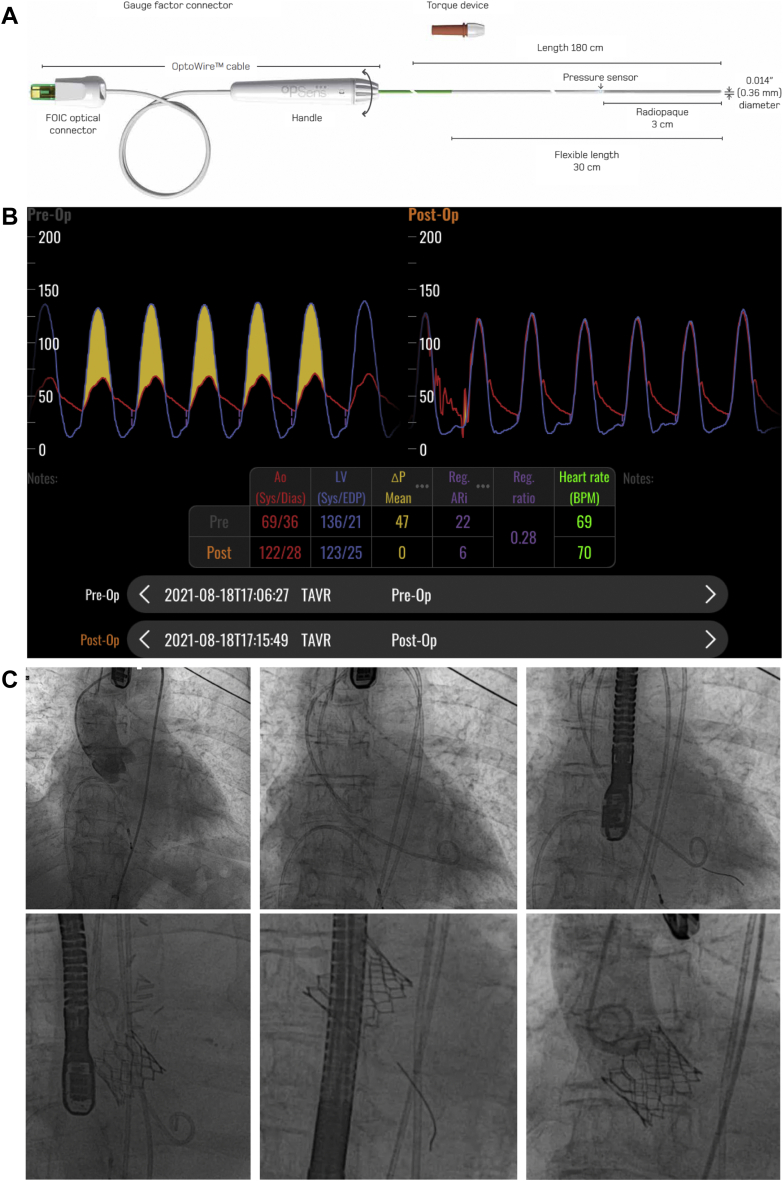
OpSens OptoWire III, monitor display, and procedure steps. (A) OpSens OptoWire III 0.014″; (B) monitor displaying pre- and post-transcatheter aortic valve replacement (TAVR) measurements; (C) summary of the procedure. Before TAVR (upper row), a root angiogram, 2-pigtail gradient, and OpSens OptoWire III-derived gradient were performed. After TAVR (lower row), 2-pigtail gradient, OpSens OptoWire III-derived gradient, and a root angiogram were performed. A transthoracic echocardiogram and a transesophageal echocardiogram were performed both before and after TAVR. FOIC, fiber optic interface connector.
